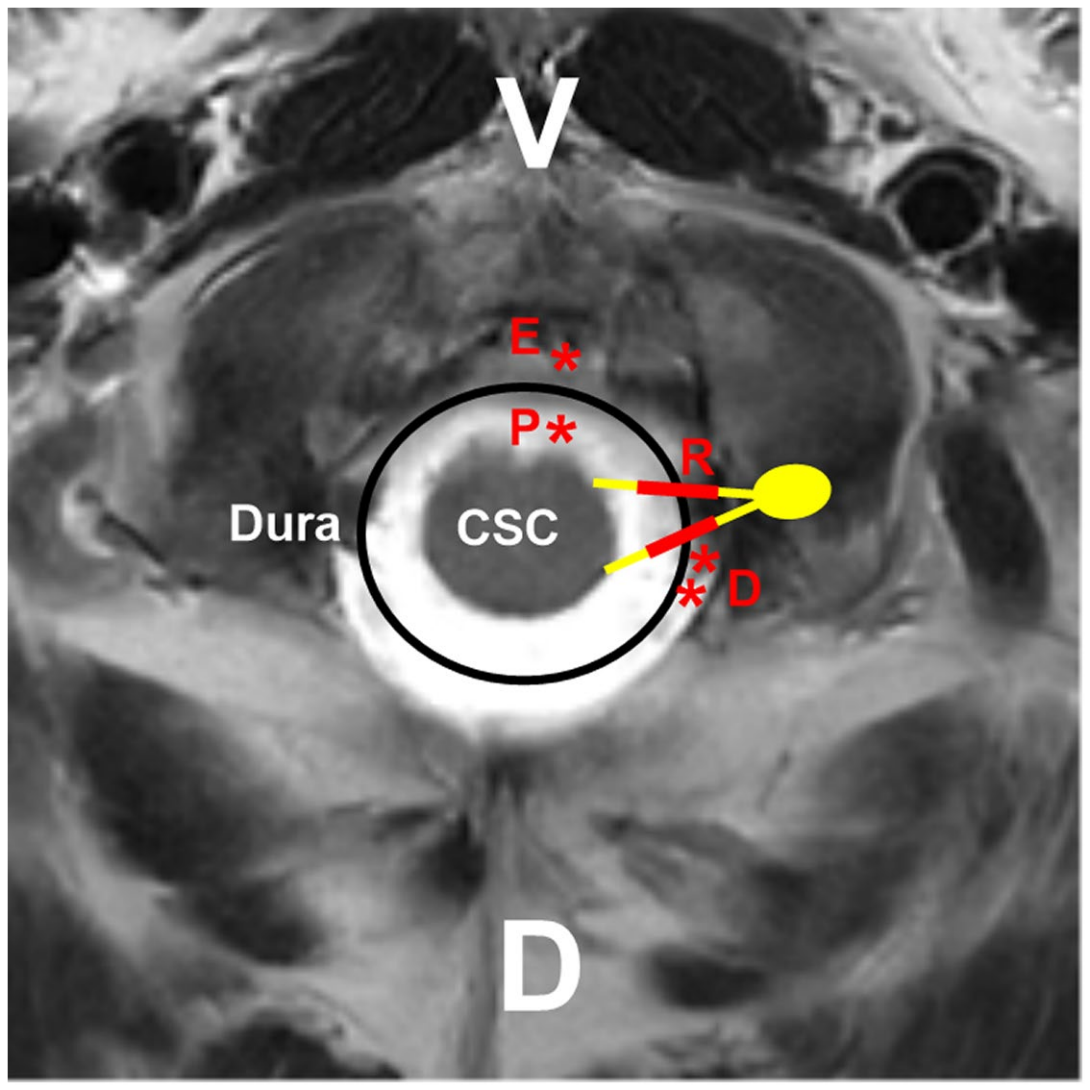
Locations of different high cervical CCJ AVFs. On the hybrid mode pattern of magnetic resonance imaging, the locations of different high cervical CCJ AVFs are shown. PAVF (red P with asterisk) located at the ventral spinal cord surface, RAVF (red R with thick line segments) located at C1-C2 nerves, DAVF (red D with double asterisks) located at the dorsal dura, EAVF (red E with asterisk) in ventral epidural space. AVF, arteriovenous fistula; C1-C2, first and second cervical vertebrae; CCJ, craniocervical junction; CSC, cervical spinal cord; D, dorsal; DAVF, dural AVF; EAVF, epidural AVF; PAVF, perimedullary AVF; RAVF, radicular AVF; V, ventral.
